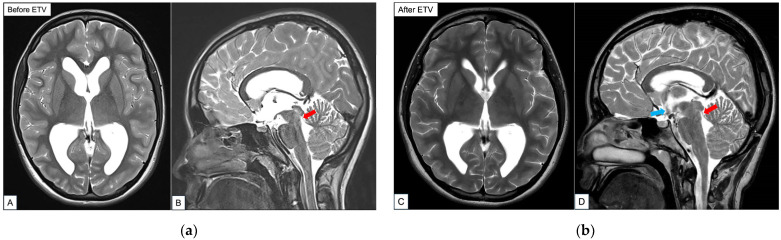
Representative T2-weighted sequence MRI brain images before ETV (a) versus after ETV (b). An objective radiological measurement of the ventricle size using the axial image is calculated via the Evans Index [9]. Briefly, this is the ratio of the maximum width of the frontal horns of the lateral ventricles and the maximal internal diameter of the skull at the same level [9,10]. (a) Pre-operative MRI brain images in axial (Image (A)) and sagittal (Image (B)) directions, depicting enlarged ventricles whereby the calculated Evans Index is 0.38. Red arrow points to a tissue web within the aqueduct of Sylvius. (b) Post-operative MRI brain images in axial (Image (C)) and sagittal (Image (D)) directions that show noticeably smaller ventricles in comparison to (a). In Image (D), there is a flow void seen (blue arrow). Here, the calculated Evans Index is 0.28. The red arrow points to a tissue web within the aqueduct of Sylvius.
